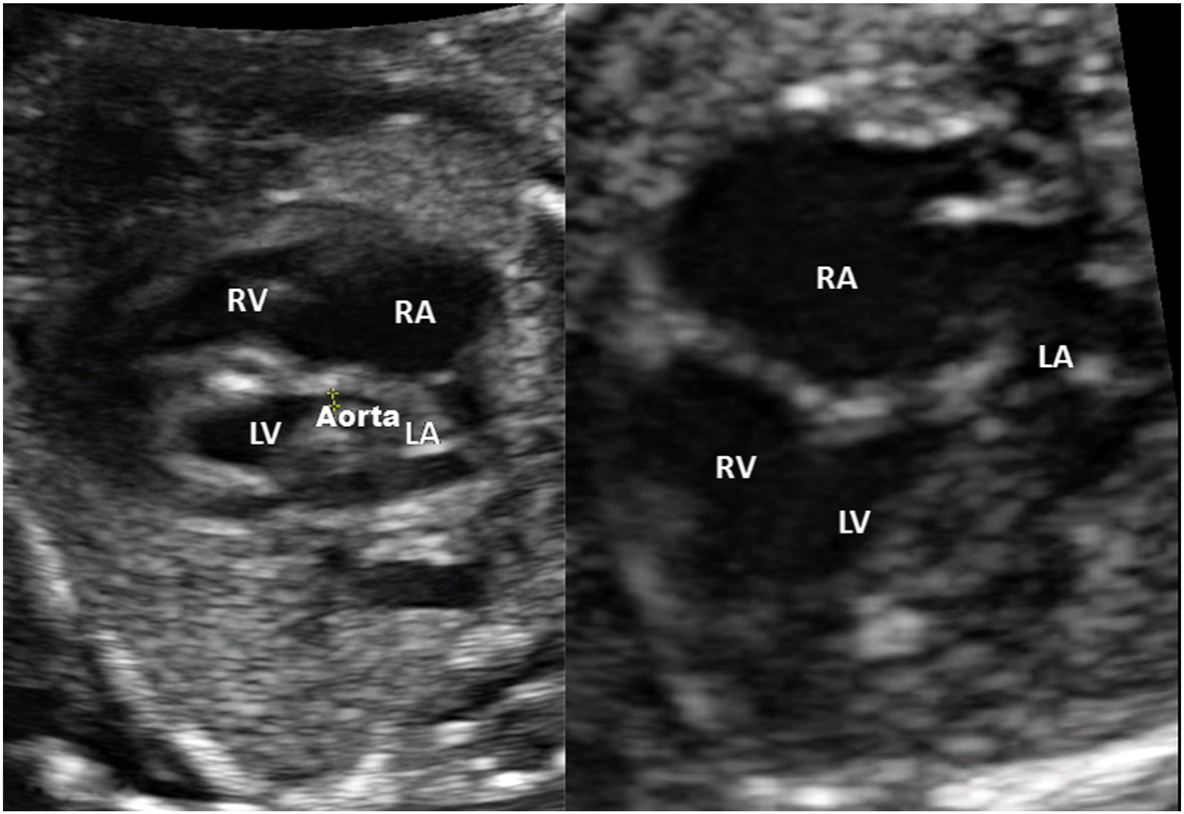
Prenatal fetal echocardiography showing a four chamber view of the fetus affected with hypoplastic left heart syndrome. Abbreviations: LA, Left atrium; LV, Left ventricle; RA, Right atrium; RV, Right ventricle.
